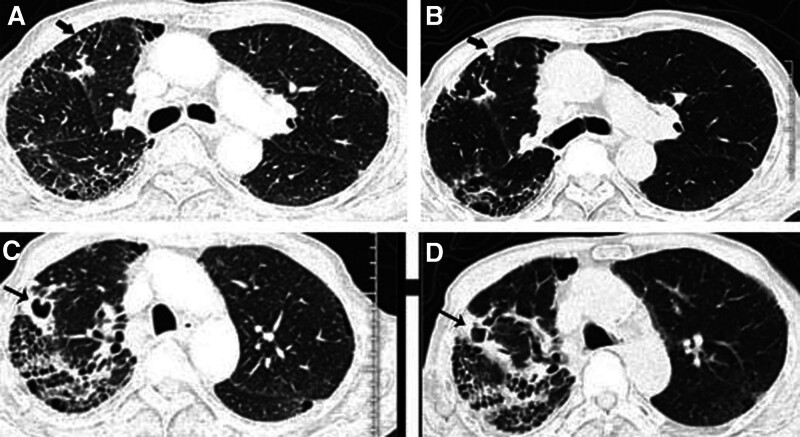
Chest HRCT reveals signs of pulmonary fibrosis, such as interstitial infiltrates with reticular patterns and areas of ground-glass opacities in the right lung. In the upper lung fields, it shows (A) a tree-in-bud pattern in the right lung (thick black arrows) and (B) centrilobular nodules at the original tree-in-bud site (thick black arrows). In the middle lung fields, (C), (D) thick-walled fibrous cavity lesions are visible (thin black arrows). HRCT = high-resolution computed tomography..
